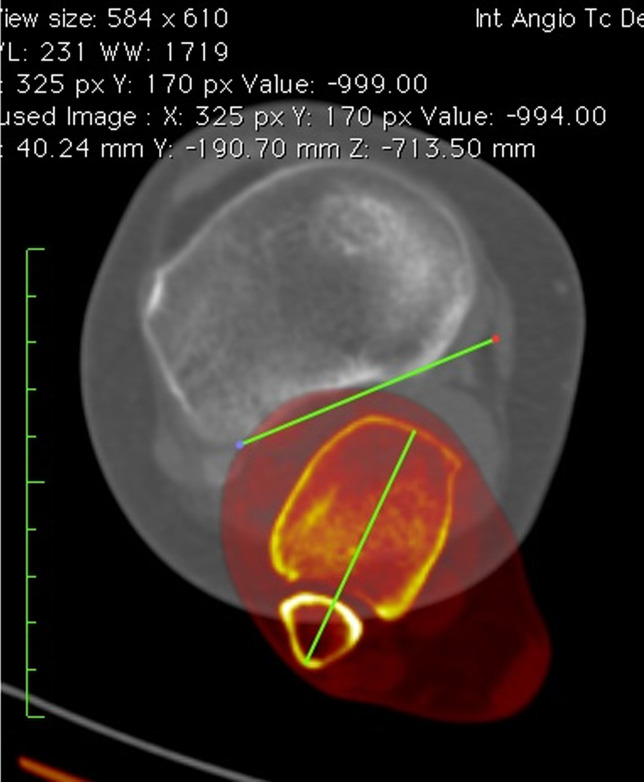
Tibial torsion. Superposition of two CT slices. The first slice is in the proximal tibia, and the second slice is in the distal tibia. Tibial torsion is measured as the angle between a line joining the posterior borders of the tibial plateaus at the level of the tibial insertion of the posterior cruciate ligament and a line joining the center of the medial and the lateral malleolus.
